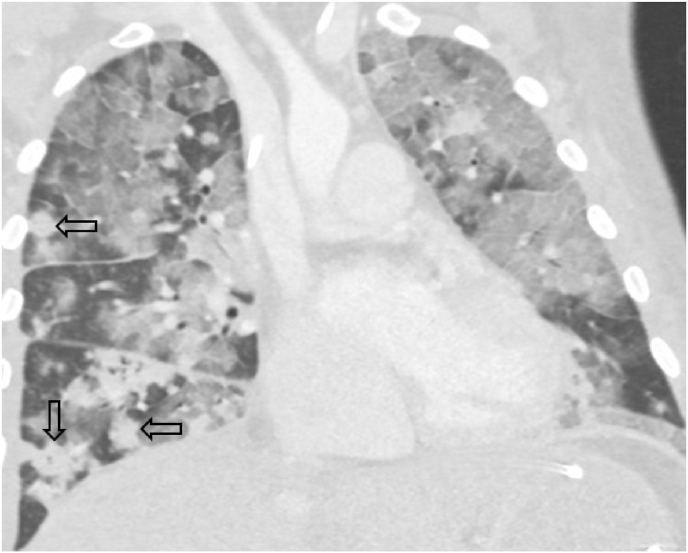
Representative coronal reconstruction image from chest computed tomography obtained following initiation of mechanical ventilation is characterized by diffuse ground-glass opacity bilaterally with intermixed nodular consolidation (arrows).
